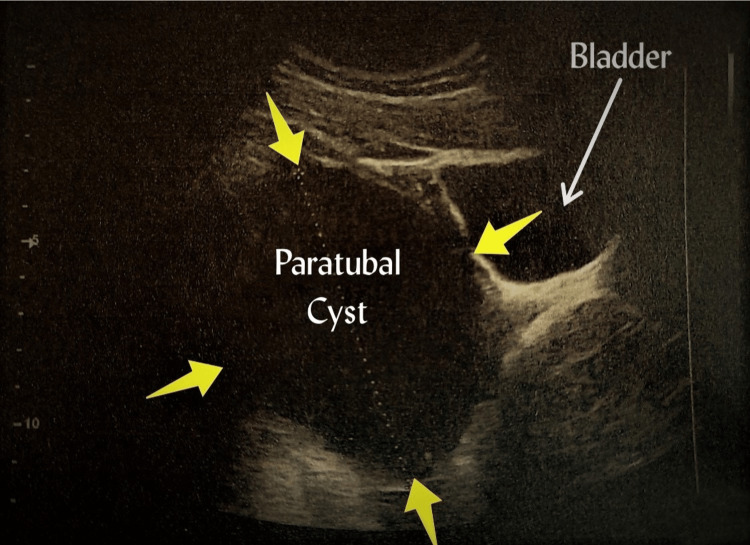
Transabdominal ultrasound imaging of a twisted paratubal cyst A unilocular, thin-walled, oval-shaped cystic formation with smooth margins in the anatomical position of the left adnexa is depicted, without simultaneous imaging of the ipsilateral ovary.
